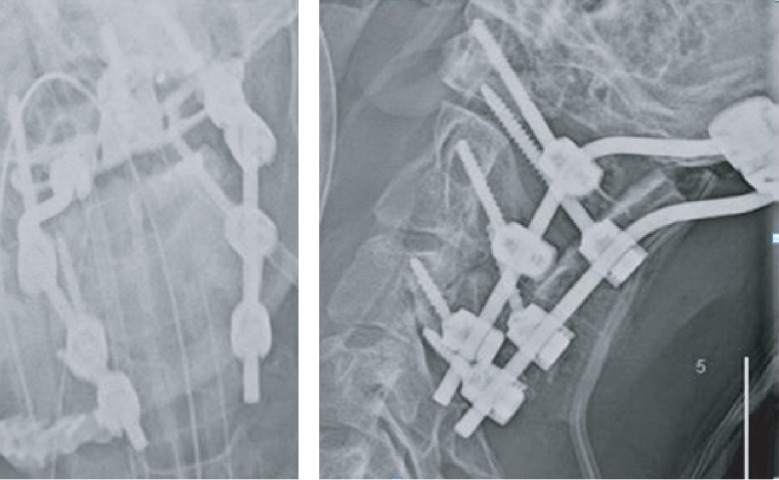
Case 2: postoperative X-ray. Occipito-C6 PSIF.
